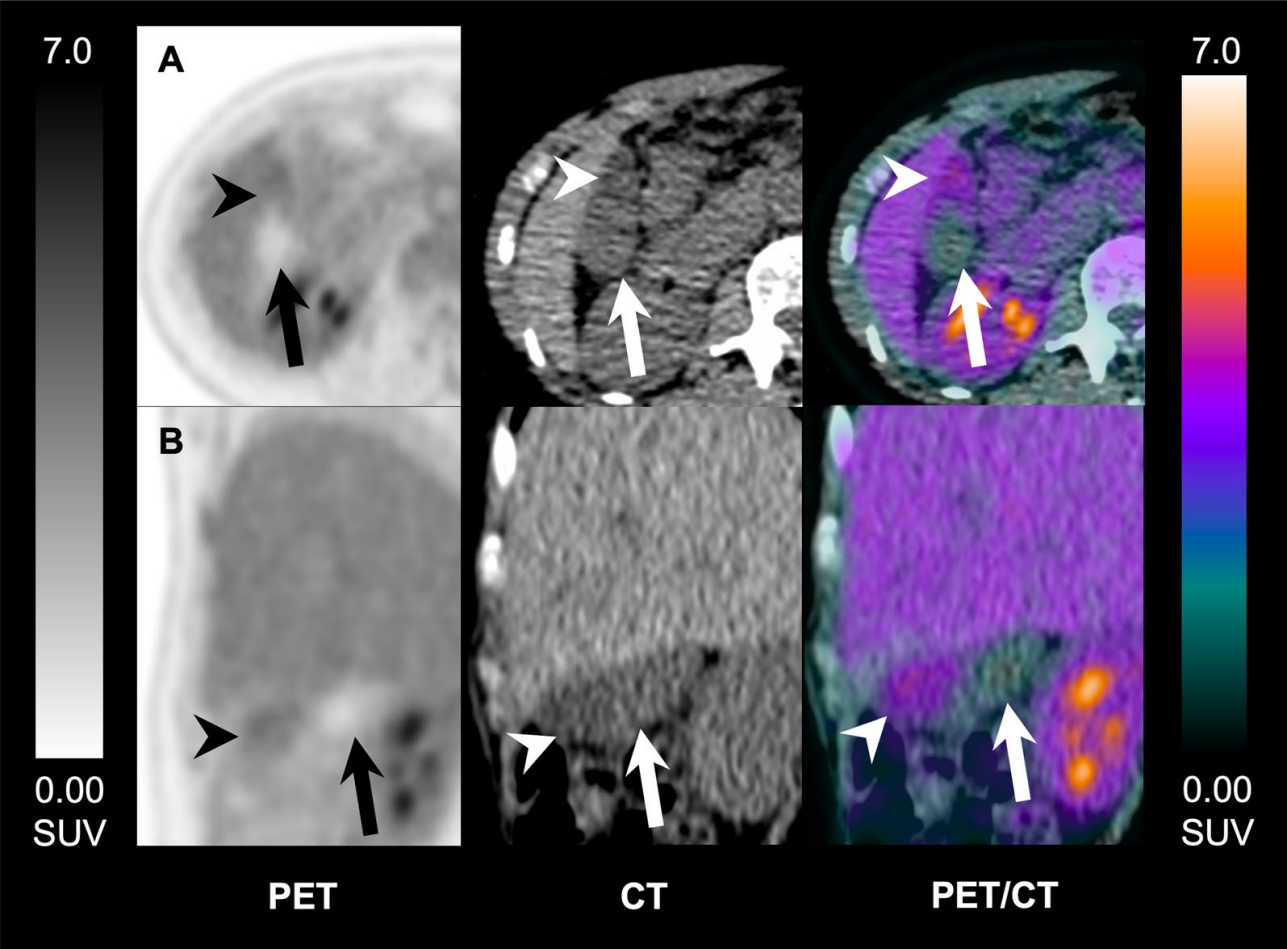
Uneven ^18^F-FDG uptake in the gallbladder. PET, CT and fused PET/CT images of a 61 y/o healthy female participant, scanned at 90-min post-injection of 334 MBq of ^18^F-FDG. Images show distribution of ^18^F-FDG uptake in the gallbladder: one portion shows uptake with lower attenuation on the corresponding CT, while the other portion shows no uptake and higher attenuation on the corresponding CT. These findings suggest the presence of different luminal content. Arrowhead: gallbladder portion showing tracer uptake with lower attenuation on the corresponding CT; arrows: gallbladder portion showing no uptake and higher attenuation on the corresponding CT. A axial, B coronal, planes of PET, CT and fused PET/CT images
